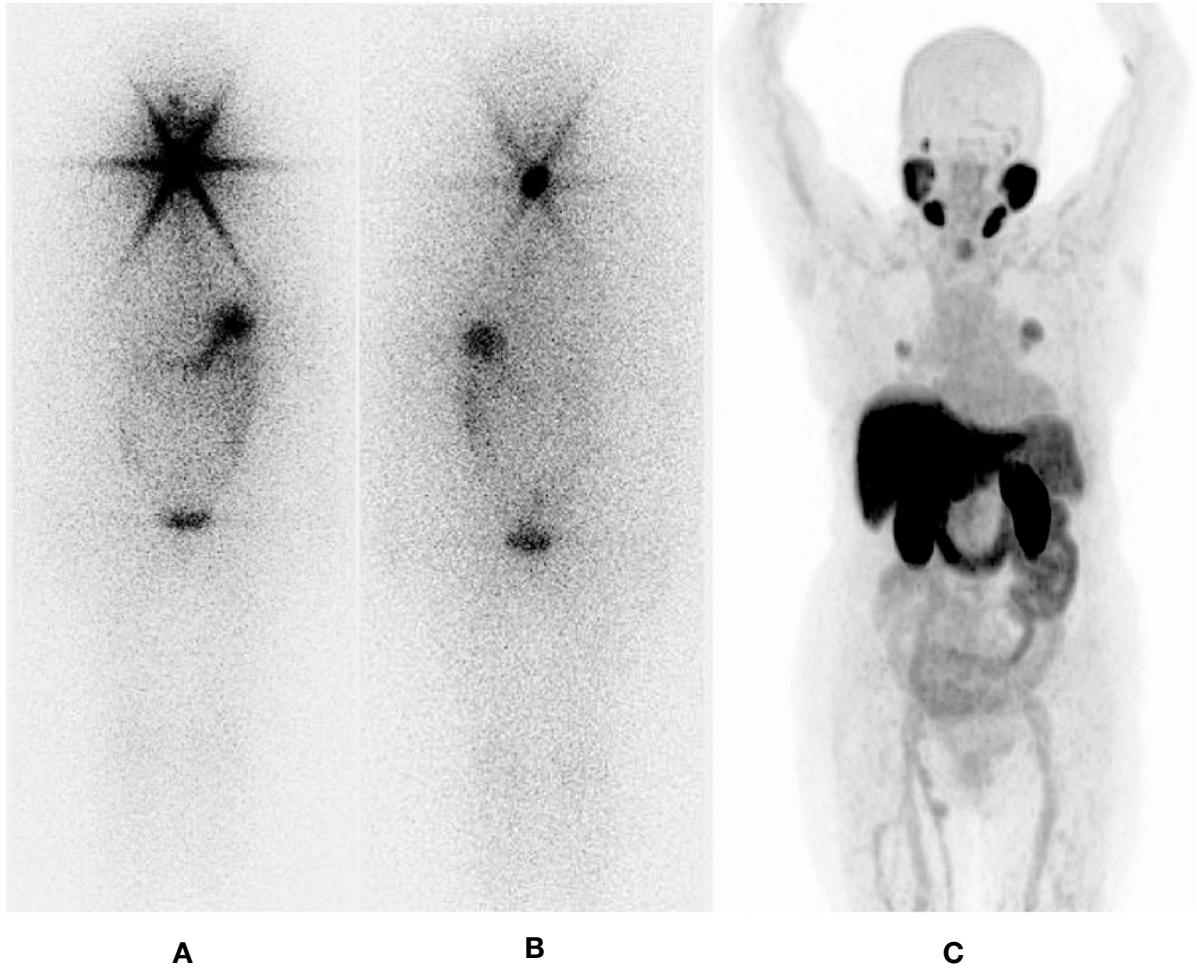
65 years old female; Thyroglobulin 253 ng/dl; treated with TT+RAI (14800 MBq). First two images show an ^131^I – post therapy whole body scan in (A) anterior and (B) posterior projections, with a star – shapped intense uptake. The image on the right (C), shows ^68^Ga – PSMA PET/CT maximum intensity projection [MIP], which manifests a glaring superiority regarding spacial resolution, and target–to–background ratio.
